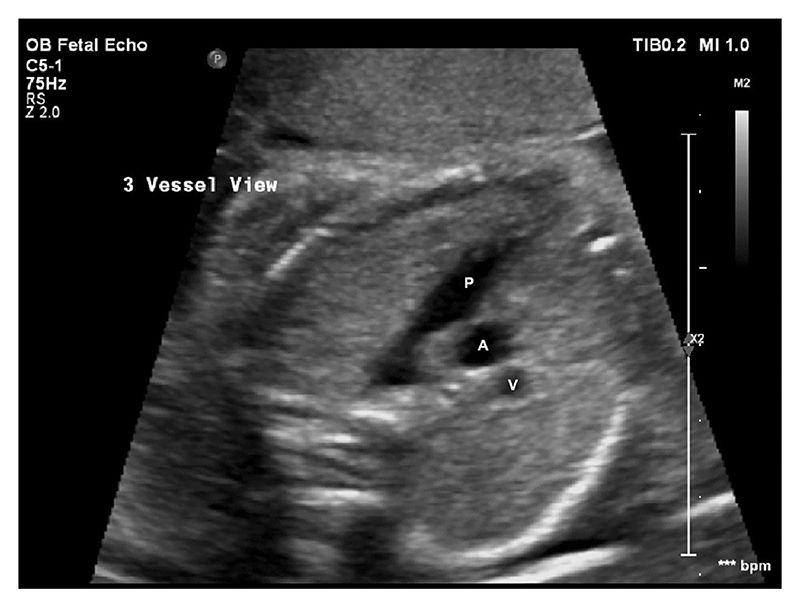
Obstetric ultrasound of fetal thorax showing the 3-vessel-view (3VV). Pulmonary Artery (P), ascending aorta (A) and superior vena cava (V).
